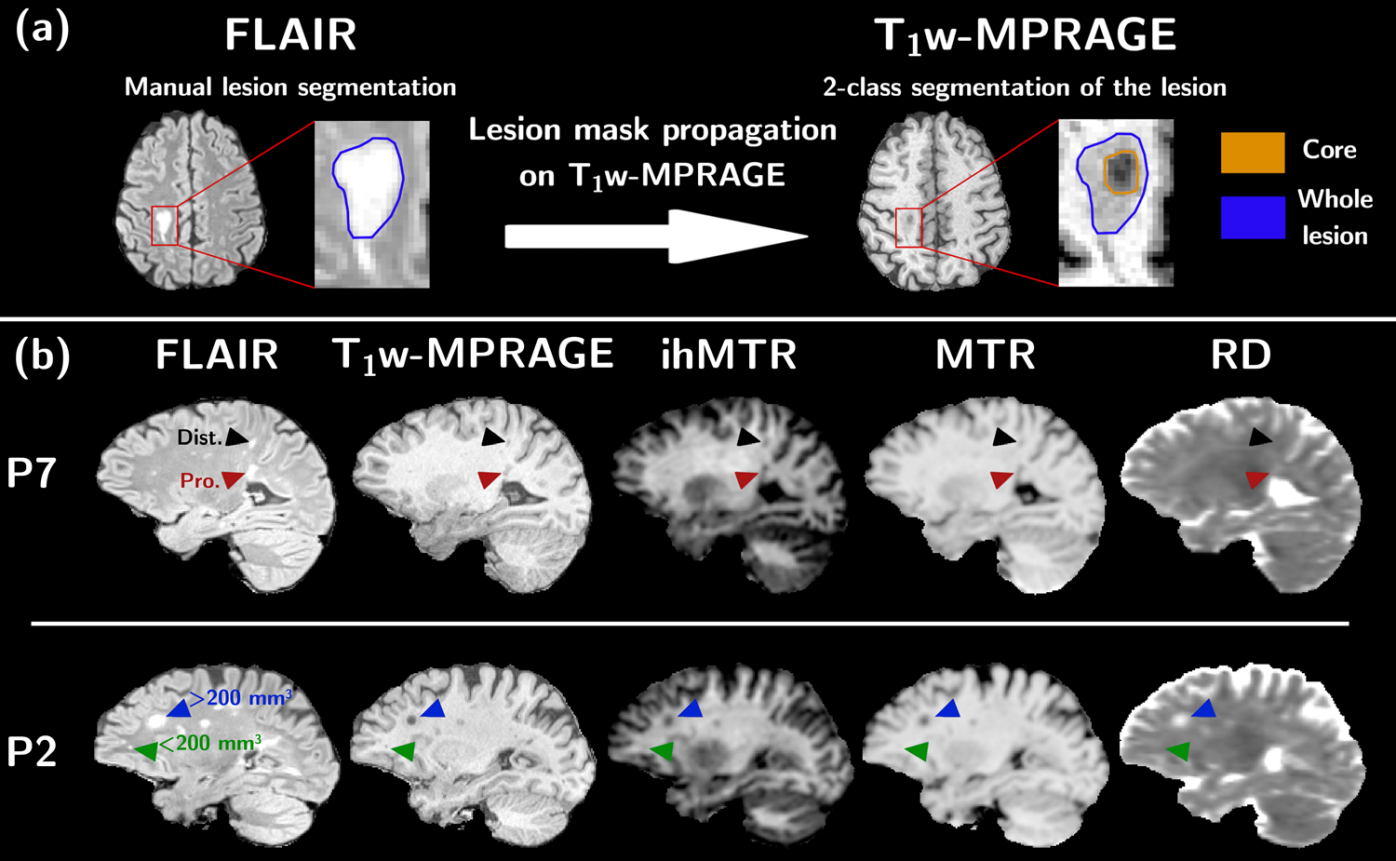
Sketch of the lesion core submask creation (a). The mask of the lesion, manually drawn on the FLAIR image, is propagated on the T_1W_-MPRAGE image. A two-class segmentation performed with the Atropos’ k-means clustering algorithm applied on the T_1W_-MPRAGE image creates the lesion core submask. Representative sagittal views of FLAIR, T_1w_-MPRAGE, ihMTR, MTR, and RD images for two different patients (b) with examples of lesions proximal (Pro., red arrows) and distal (Dist., black arrows) from the ventricles (patient P7), as well as large (> 200 mm^3^, blue arrows) and small (< 200 mm^3^, green arrows) lesions (patient P2).
